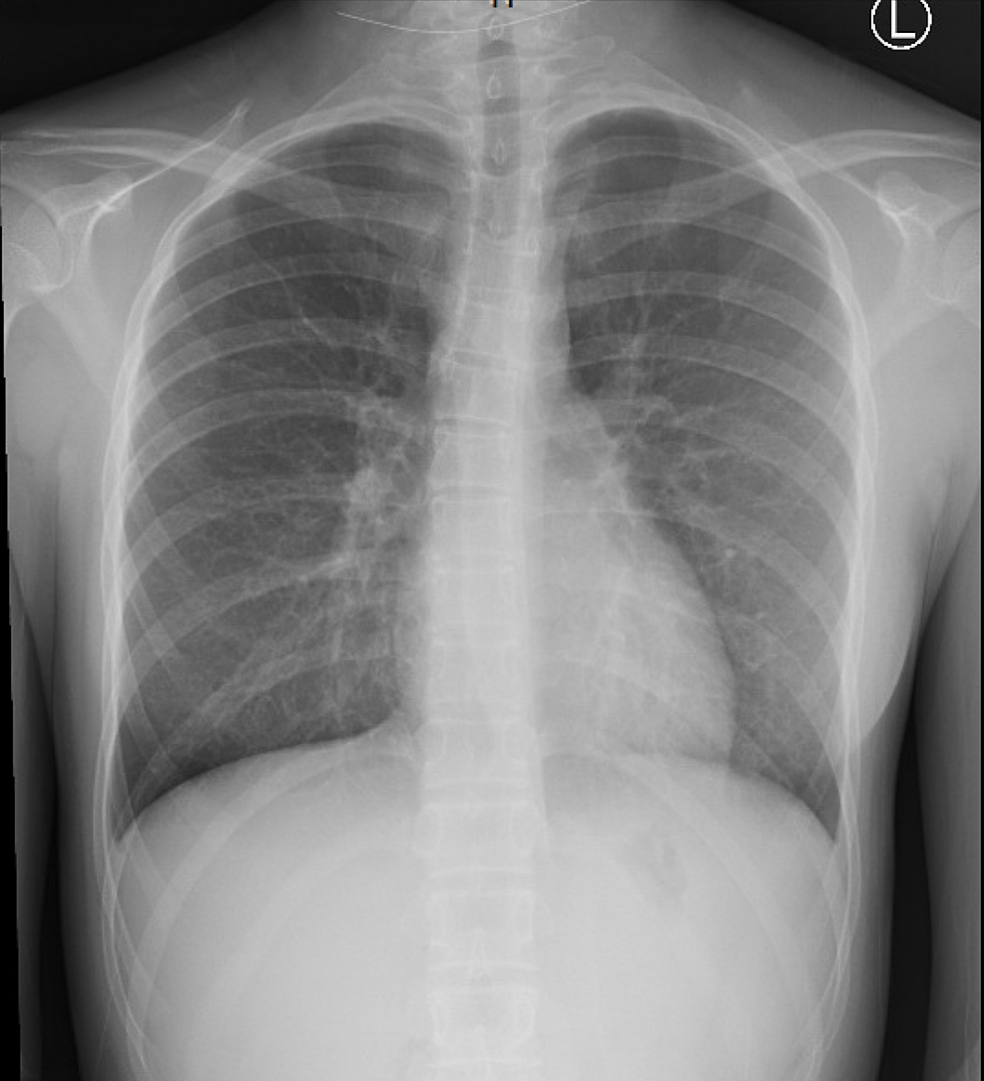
CXR showing mild hyperinflation with prominent interstitial markings CXR: chest X-ray
